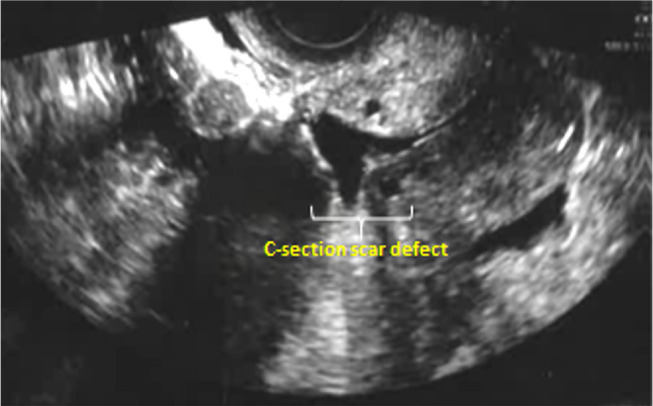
Transvaginal ultrasound examination showing a C- section scar defect.
